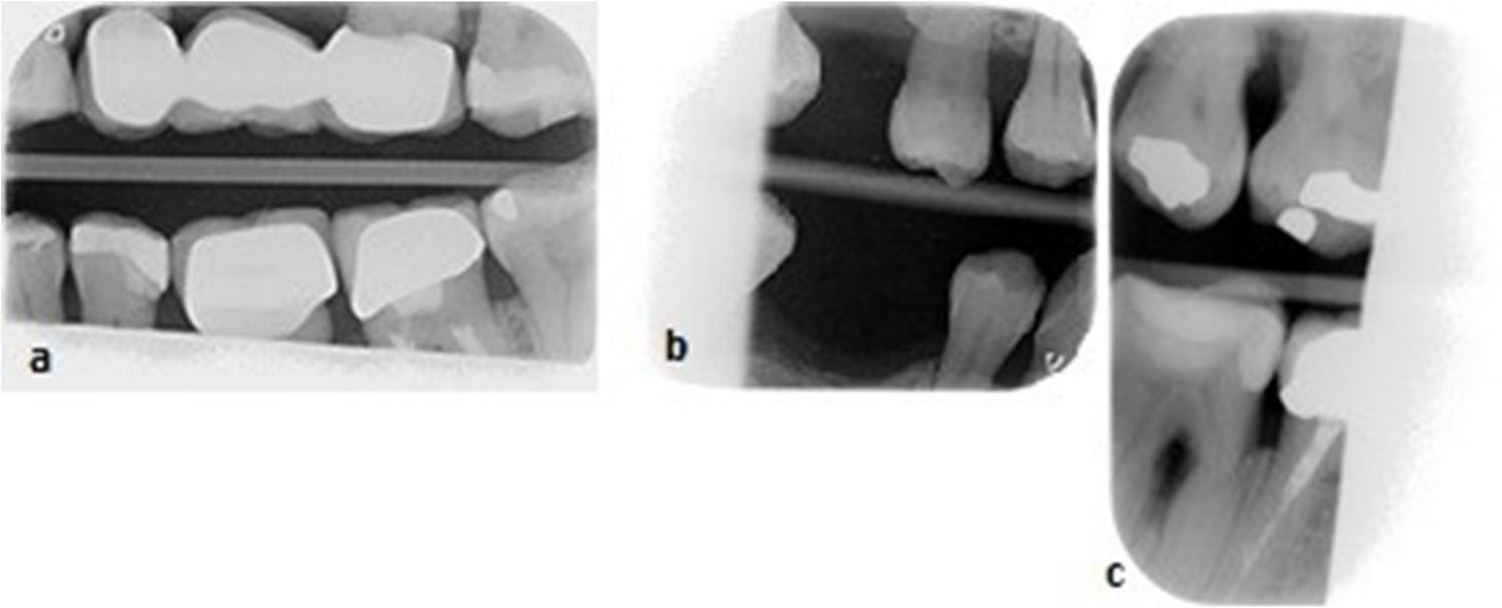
Bitewings with cone cutting that affects the diagnostic quality (score 2). The bone level next to the teeth was not visible at more than one site due to cone cutting (a). The number of missing teeth was more than one due to cone cutting (b). The number of missing contacts of approximal surfaces was more than one due to cone cutting (c)
